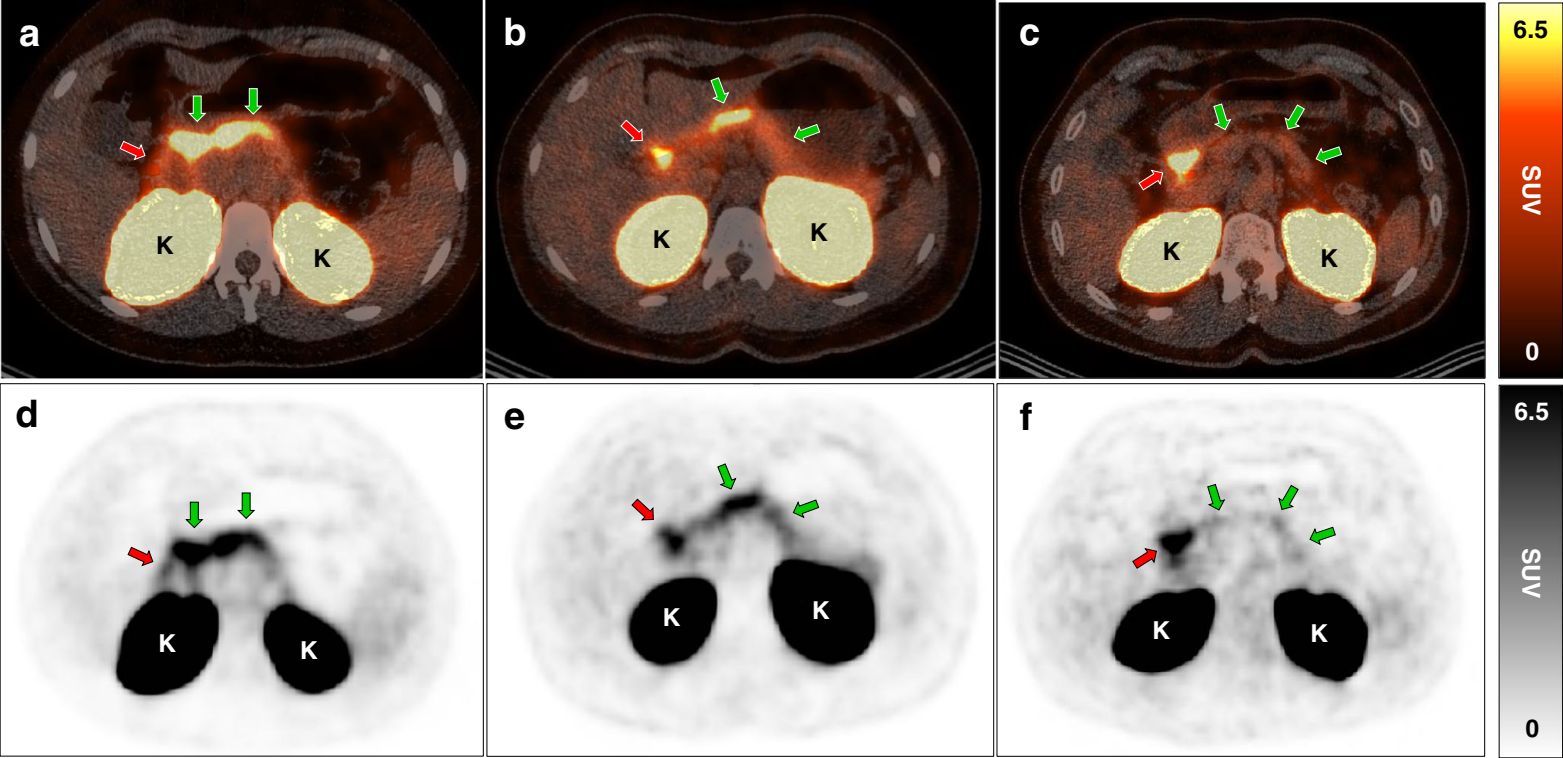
Abdominal PET/CT images with pancreatic uptake of radiolabelled exendin. Transversal fused PET/CT images (a–c) and PET images (d–f) of three individuals showing pancreatic uptake of ^68^Ga-exendin as measure for beta cell mass (green arrows). Other regions with exendin uptake are the proximal duodenum (red arrows) and the kidneys (indicated with the letter ‘K’). Pancreatic exendin uptake of individuals with LGV were in the same range for individual 1 (a, d) (AUC for C-peptide 122 nmol min/l) and individual 2 (b, e) (no detectable C-peptide), despite differences in C-peptide response, and much greater than in individual 3 (c, f) (no detectable C-peptide)
